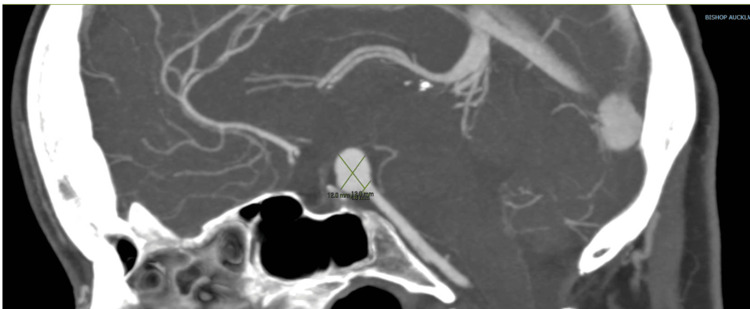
Represents CT brain of 12x12x4 mm basilar tip aneurysm on sagittal plane view
